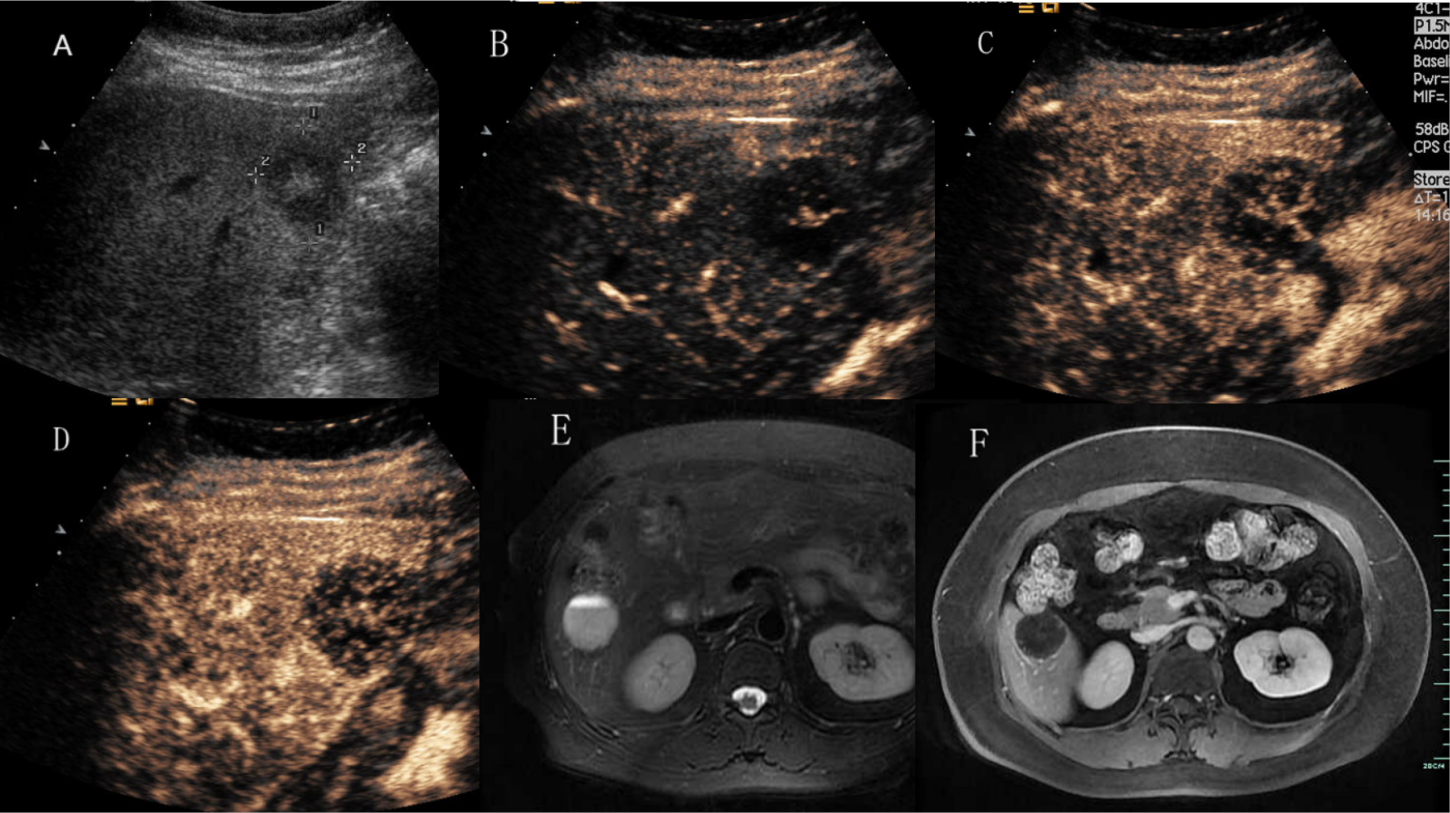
Hepatic hemangioma with slowly spoke wheel enhancement (A) Ultrasound revealed a hypoechoic mass in hepatic segment VI. (B), (C) and (D) CEUS showed slow spoke-wheel enhanced pattern. (E) T2-weighted MR images revealed a high signal intensity lesion with fluid–fluid level. (F) Contrast enhanced MR images showed slightly rim enhanced.
